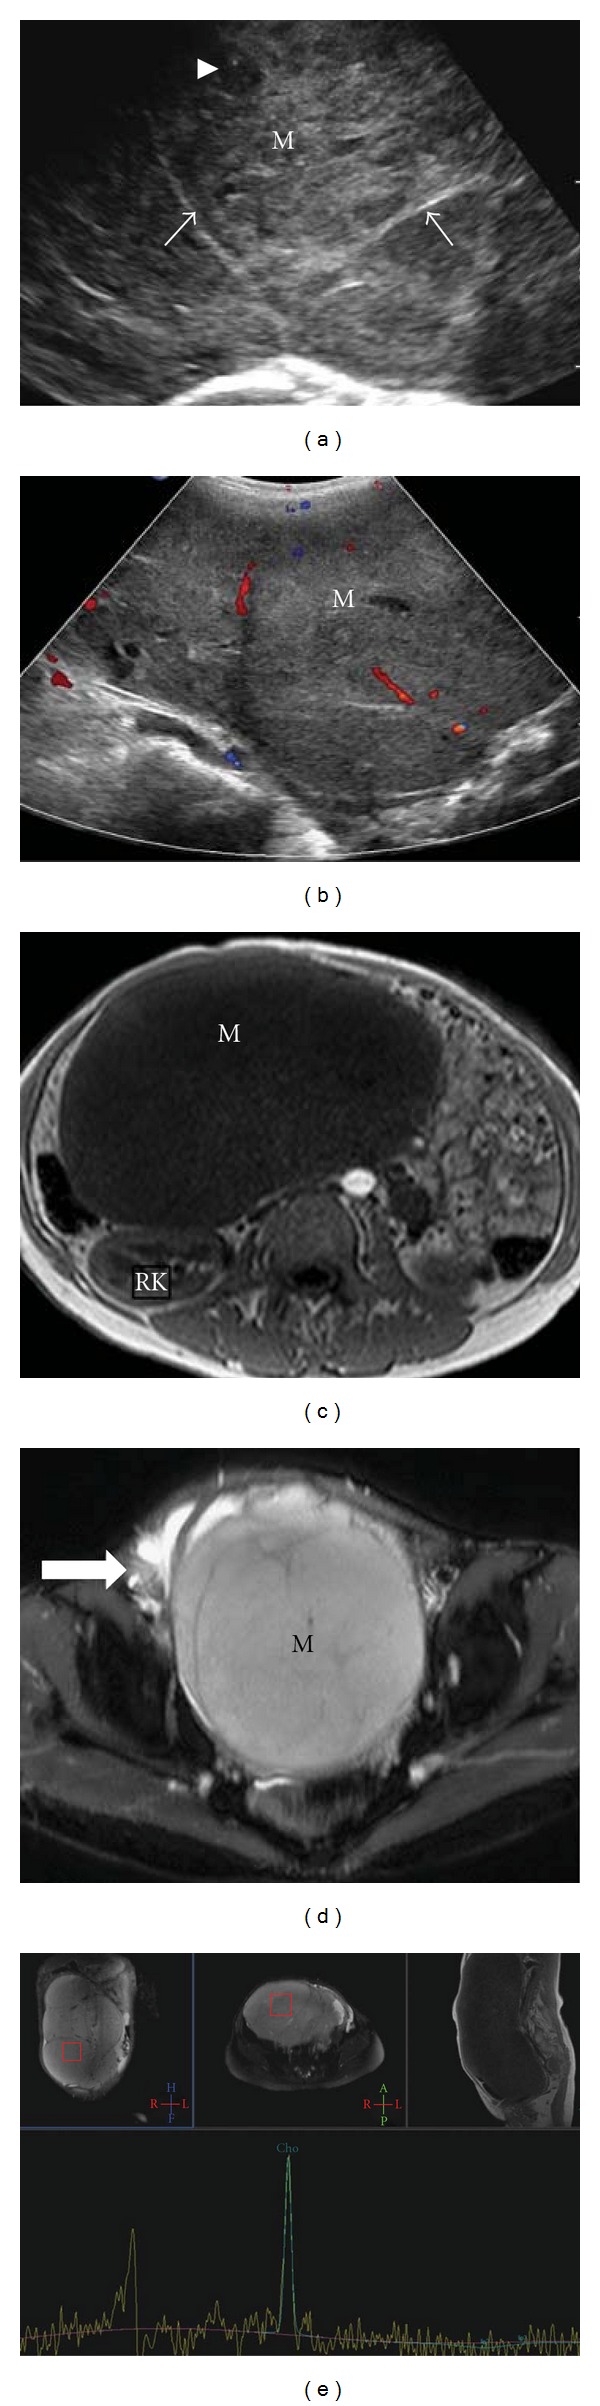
(a) Transabdominal ultrasound showing a heterogeneously hypoechoic mass (M) with internal septations (white arrows) and central necrosis (arrowhead). (b) Color and spectral Doppler images showing few internal vascular channels with arterial flow. (c) T1W axial image of the abdomen. The tumor is hypointense causing mass effect on to the adjacent structures. The right kidney is pushed more posteriorly. (d) T2W axial image showing the tubular gonadal structure in the region of right inguinal canal. (e) MR spectroscopy showing a high choline peak compatible with a tumor spectrum.
